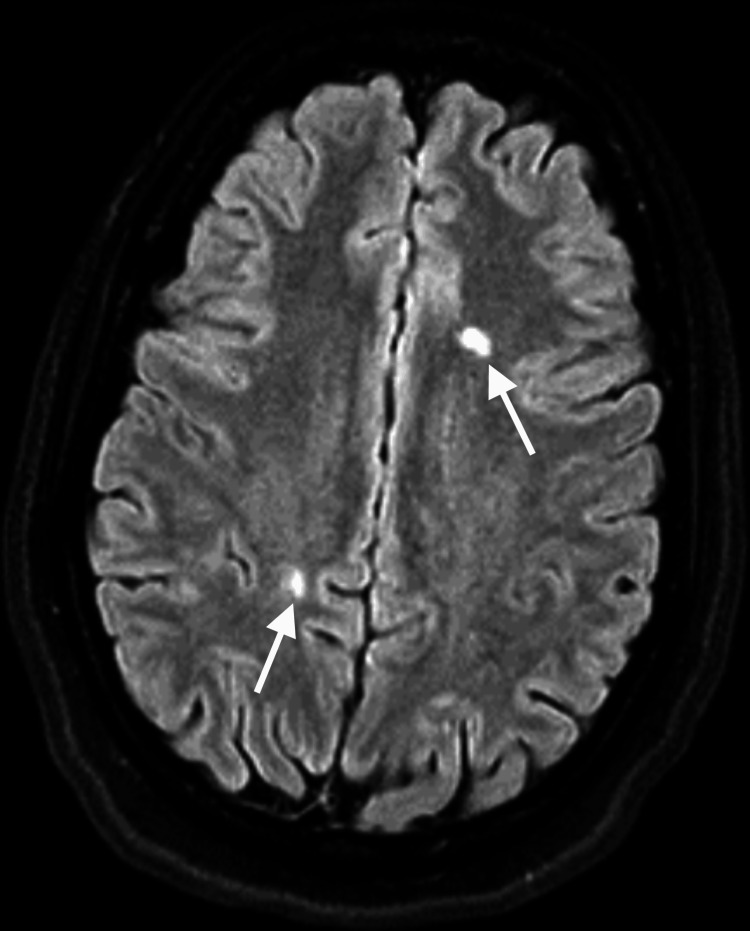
Postictal brain MRI (axial section, FLAIR) Two roughly oval-shaped lesions, in hypersignal FLAIR, with their long axis perpendicular to the ventricular axis, affecting the centrum semiovale MRI: magnetic resonance imaging; FLAIR: fluid-attenuated inversion recovery
